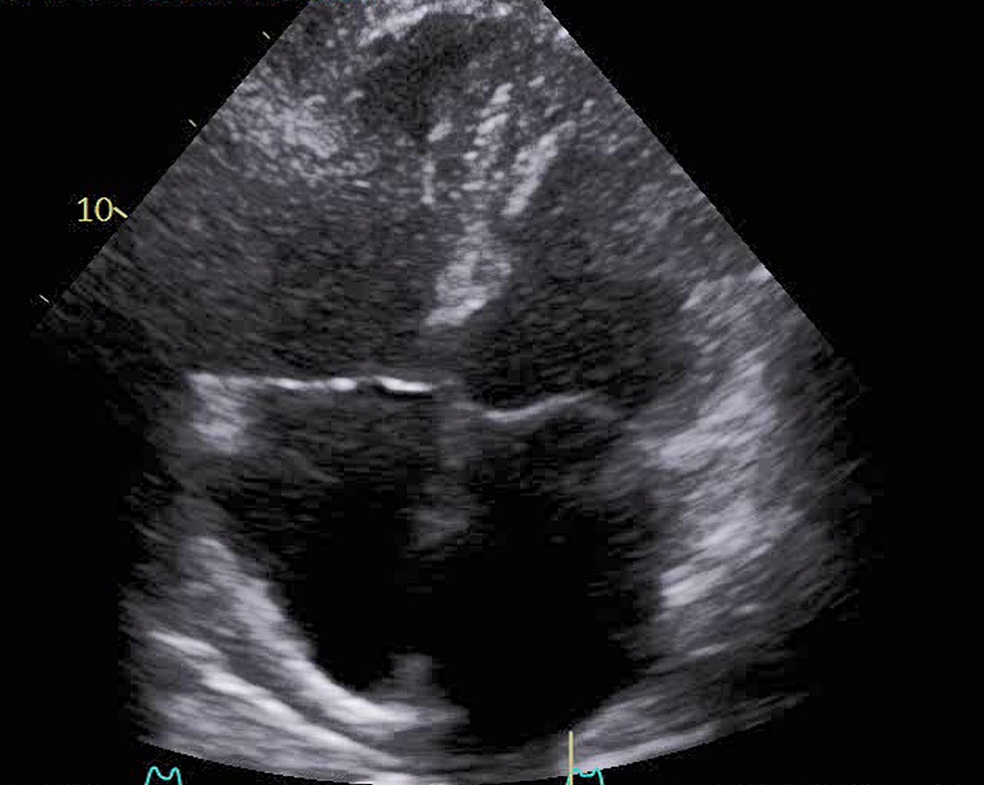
Limited echocardiogram following tPA therapy showing complete resolution of the intracardiac mass tPA: tissue plasminogen activator
